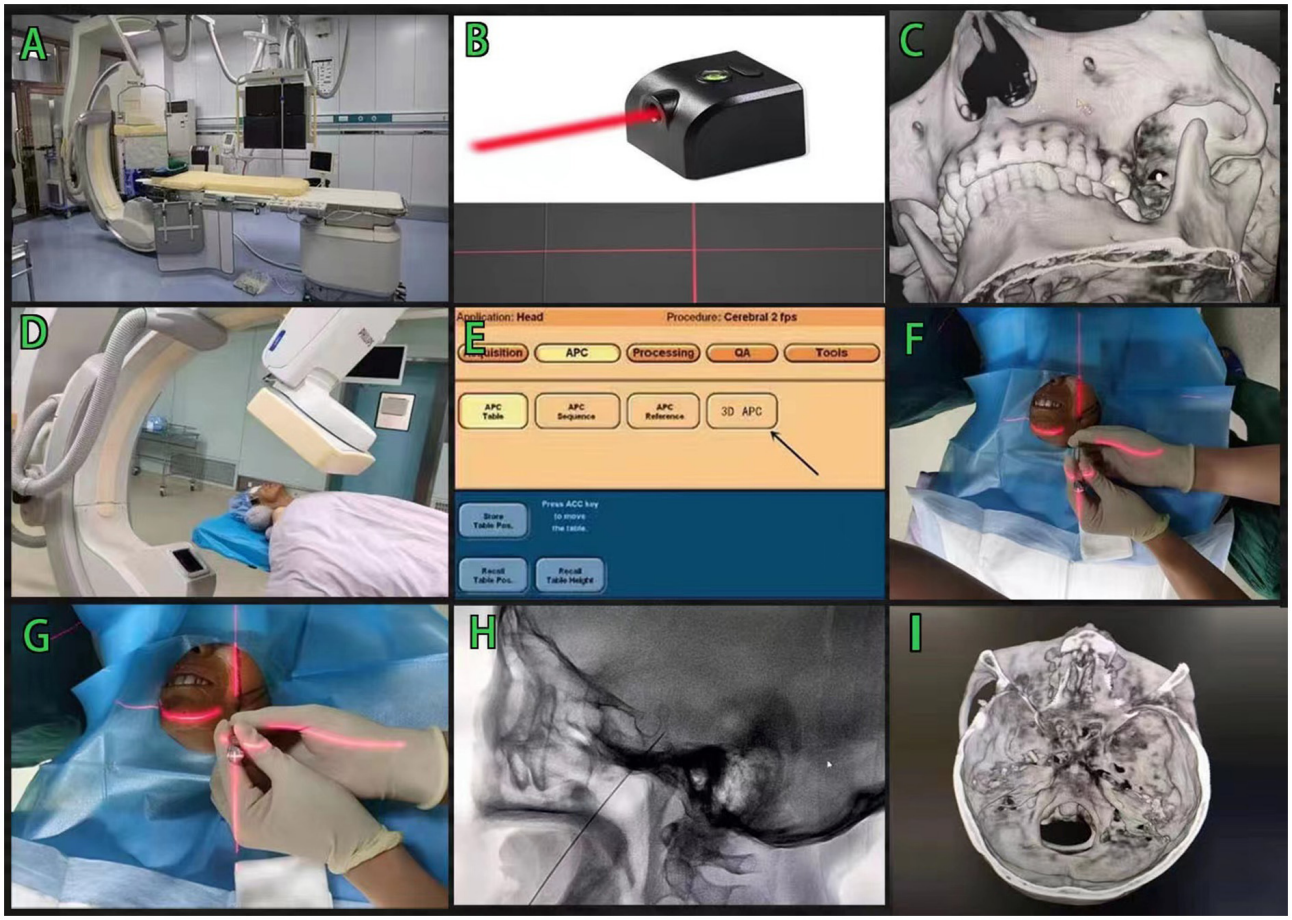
Laser navigation assisted DSA radiofrequency thermocoagulation in the treatment of trigeminal neuralgia. (A,B) Philip FD20 Digital Subtraction Angiography machine and cross-laser transmitter, (C) Adjust the 3D image so that the development line overlaps with the oval foramen, (D,E) Use the 3DAPC function in the Xper-APC automatic position control module to move the rack to a specified angle, (F,G) The puncture needle is punctured in the direction of the cross laser, and (H,I) The lateral X-ray of the head was taken to verify that the tip of the needle was in the oval foramen.
